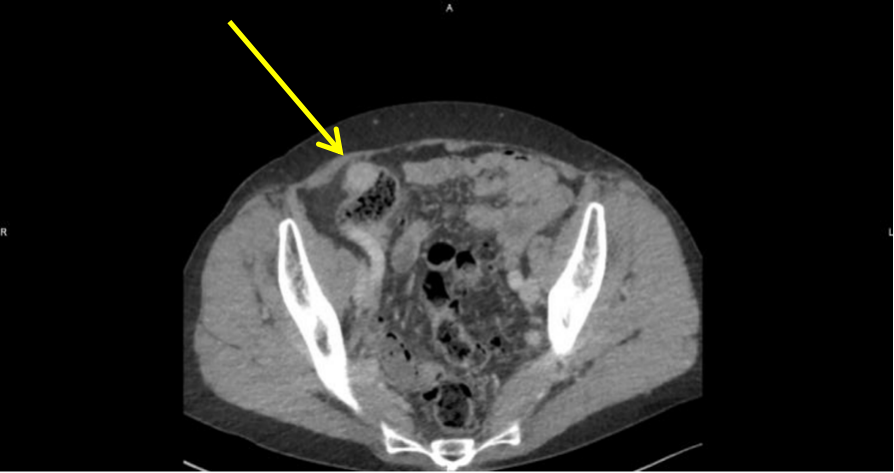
CT scan (axial) showing the hypervascular well-circumscribed cecal mass
